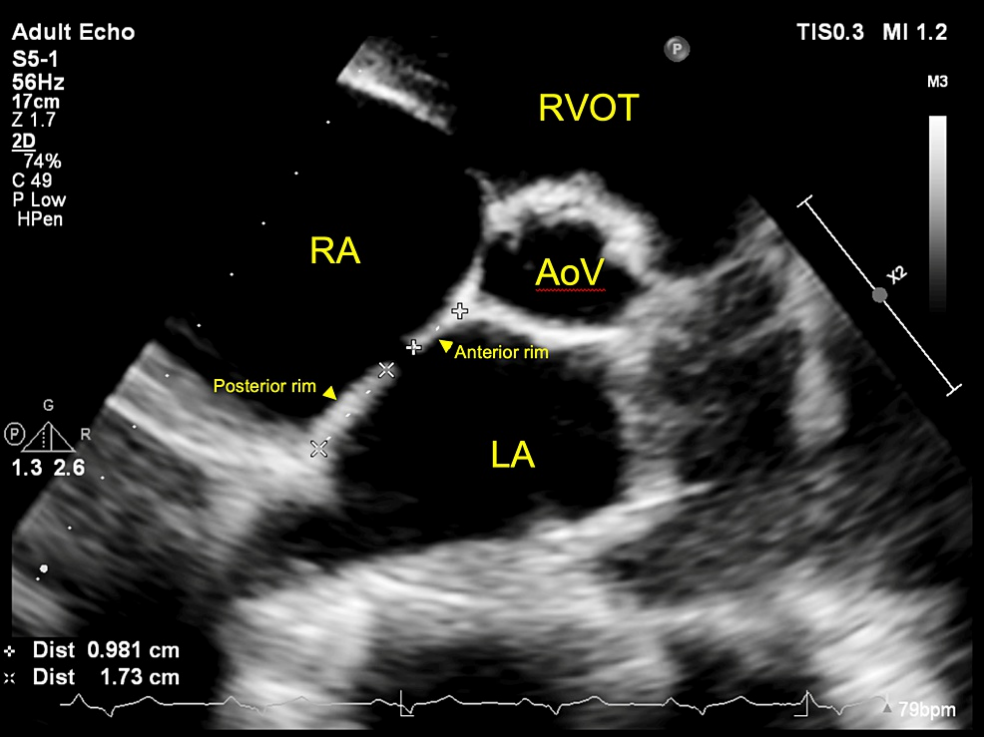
Two-dimensional TTE, parasternal short-axis, aortic valve level, demonstrates the measured anterior and posterior ASD secundum defect. TTE: Transthoracic echocardiography; ASD: Atrial septal defect.
